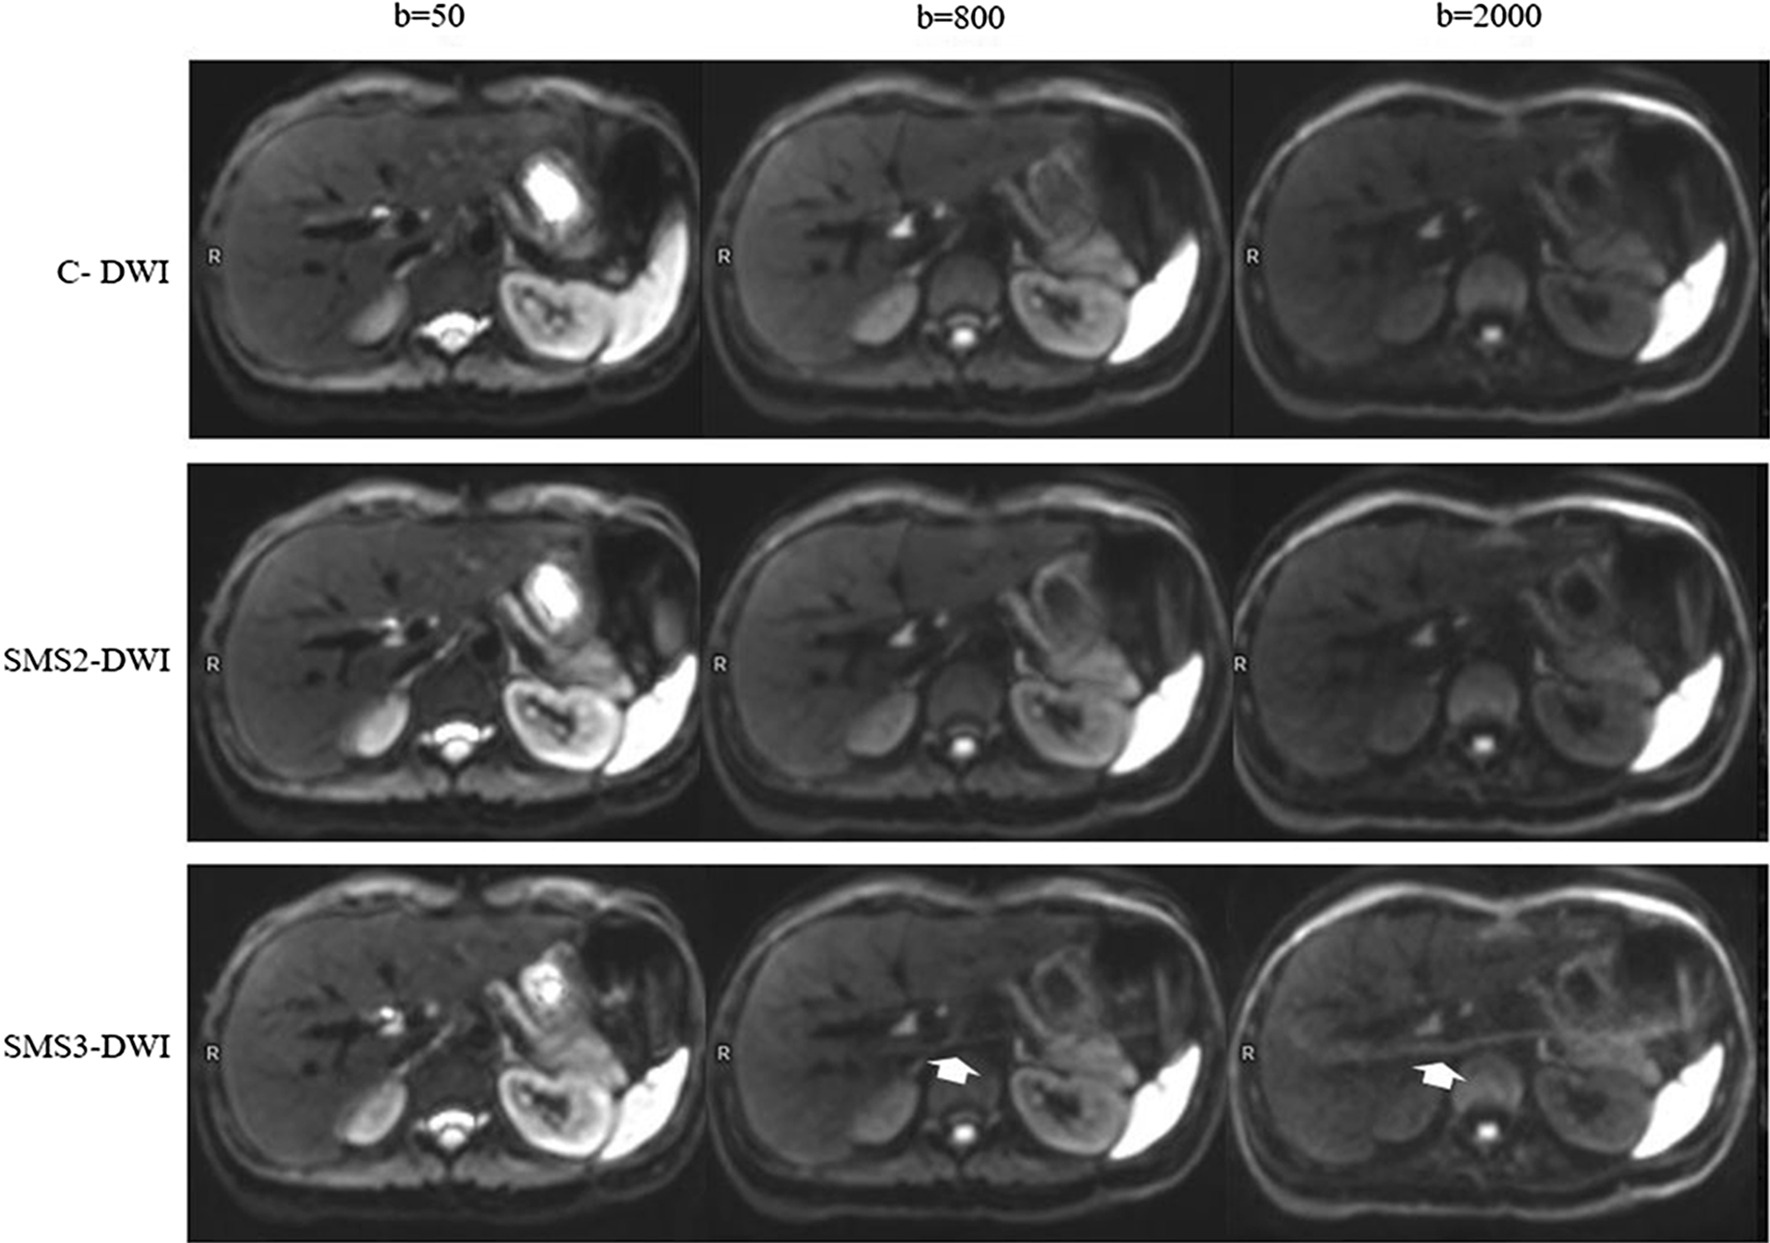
Comparisons of image quality of conventional DWI and SMS-DWI with different acceleration factors. Images were obtained in a 26-year-old healthy male volunteer with b values of 50, 800, and 2000 s/mm^2^ using SMS2-DWI, with acceleration factors of 2 (SMS2-DWI) and 3 (SMS3-DWI), and the conventional DWI sequence was used as a reference. The image quality of SMS2-DWI was rated as high as that of conventional DWI. In SMS3-DWI, substantially reduced image quality was found with signal loss in the central image portions as well as pronounced artefacts (white arrow), especially in the higher b-value images
